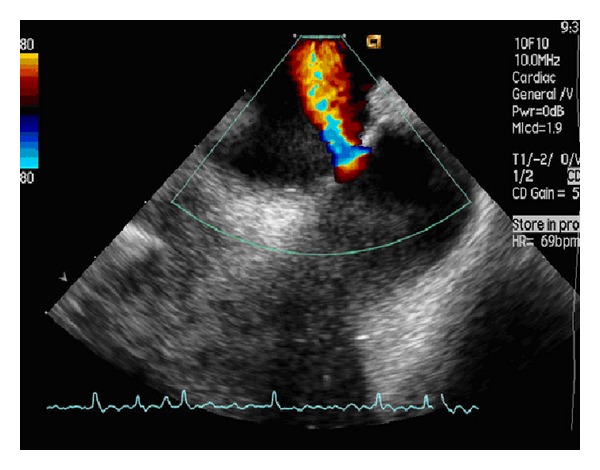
Colour Doppler imaging during intracardiac echocardiography. Flow through interatrium septum is seen via Doppler imaging, excluding iatrogenic perforation of atrium wall and confirming correct positioning of transseptal catheter into the LA.
